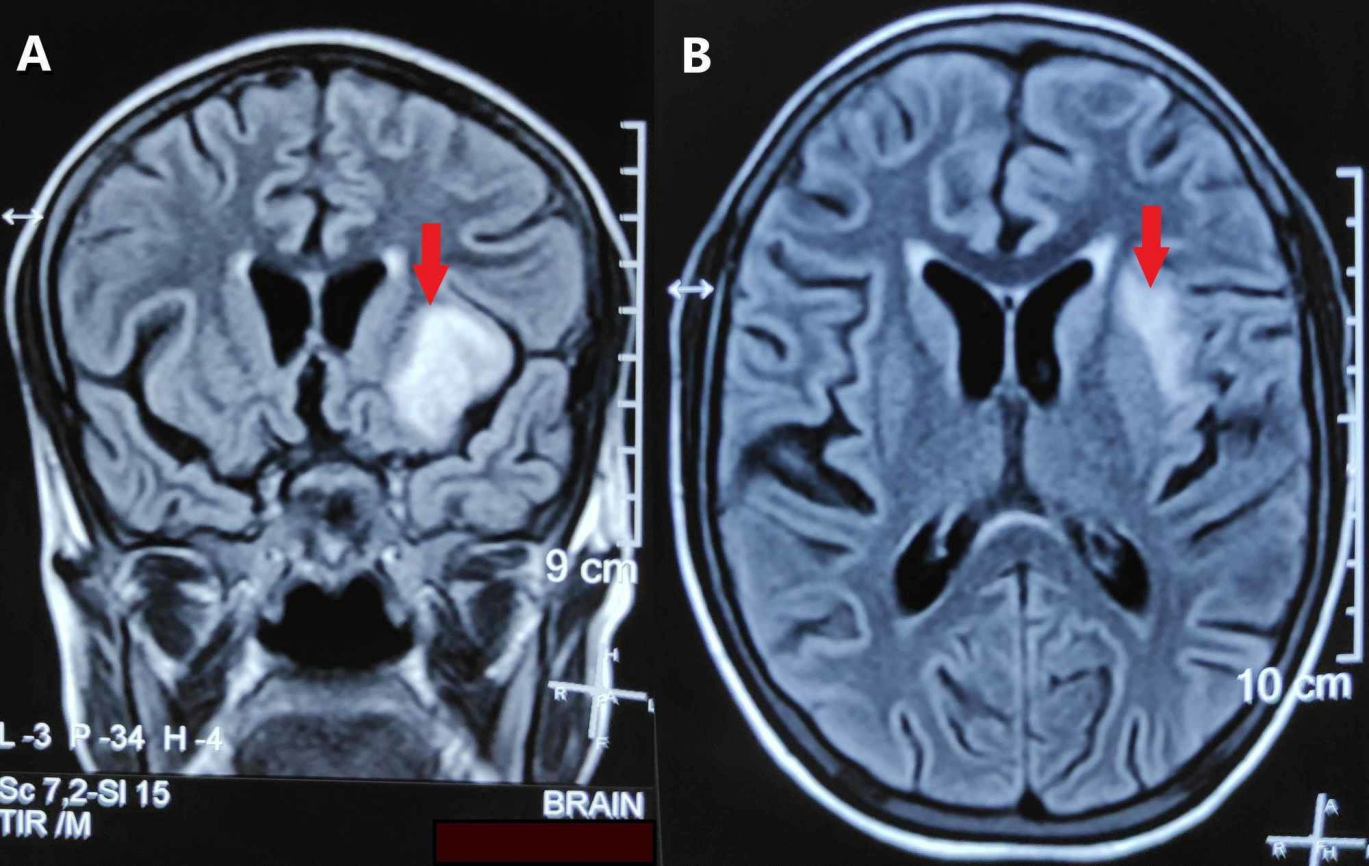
Coronal (A) and axial (B) sections of FLAIR sequence of MRI brain showing hyperintensity (red arrows) in left temporal lobe, suggestive of focal cerebritis. FLAIR, fluid-attenuated inversion recovery; MRI, magnetic resonance imaging.
